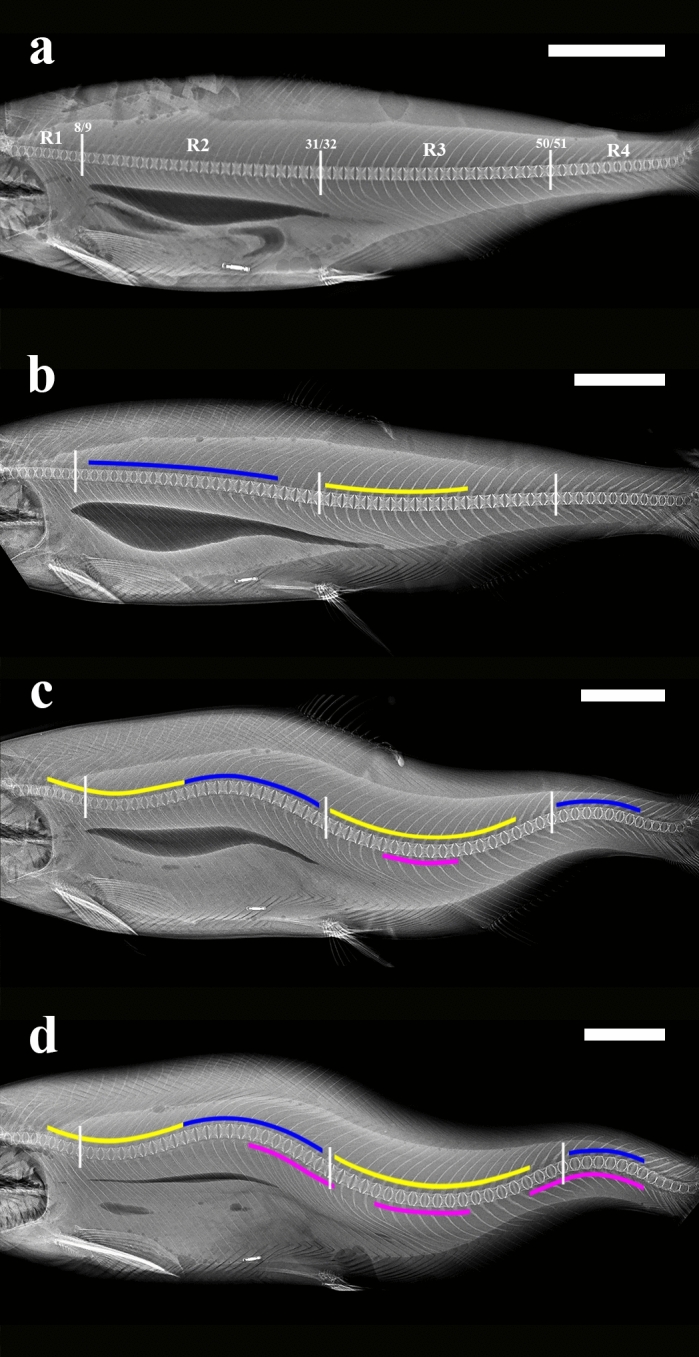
Progression of spinal curvature development evident on lateral radiographs of a Study II individual at assessments 5 (a), 11 (b), 13 (c) and 15 (d) months post-seawater transfer. Labels R1, R2, R3 and R4 in (a) correspond to spinal regions 1 (vertebra (V)1–8), 2 (V9–31), 3 (V32–50) and 4 (V51–62 +). Small vertical white lines in (a)–(d) indicate the transition points between each of the four spinal regions. Coloured lines indicate lordosis (yellow), kyphosis (blue) and scoliosis (pink). Scale bars = 5 cm. (a) 5 months post-seawater transfer, pre-radiographic curvature onset. All vertebrae exhibit the regular “X in a box shape”, are evenly spaced, and are dorso-ventrally aligned with one another in all regions. The vertebral column exhibits no angular deviation indicative of spinal curvature. (b) 11 months post-seawater transfer. Radiographic curvature onset has occurred, with kyphosis present in R2 and lordosis in R3. R1 and R4 are unaffected. (c) 13 months post-seawater transfer, post-radiographic curvature onset. Lordosis is now present in R1 as well as R3, and kyphosis in R4 in addition to R2. The angle of curvature of the existing lordosis in R2 and kyphosis in R3 has increased, and scoliosis has developed in R3 at the apex of the lordotic curve in this region. The individual now exhibits a pattern of alternating lordosis and kyphosis down the length of the vertebral column. (d) 15 months post-seawater transfer (harvest), end-stage curvature phenotype. The angle of curvature has increased for all existing curvatures, and scoliosis has developed in R2 at the transition zone between the kyphosis in R2 and lordosis in R3, and R4 at the apex of the existing kyphotic curve.
